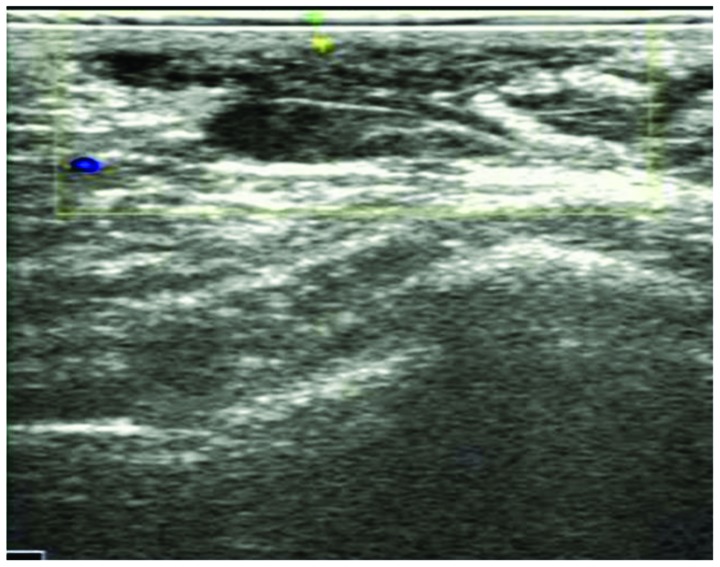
Breast ultrasonography revealing the subdermal location of the tumor, its adherence to the skin and its invasion of the subcutaneous tissue layer. No significant blood flow was observed around or within the tumor.
